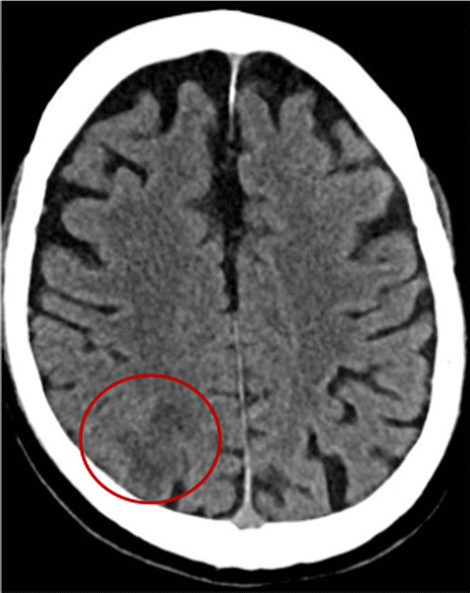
Non-contrast computed tomography (CT) scan performed in the emergency department showing a right parietal hypodense area
